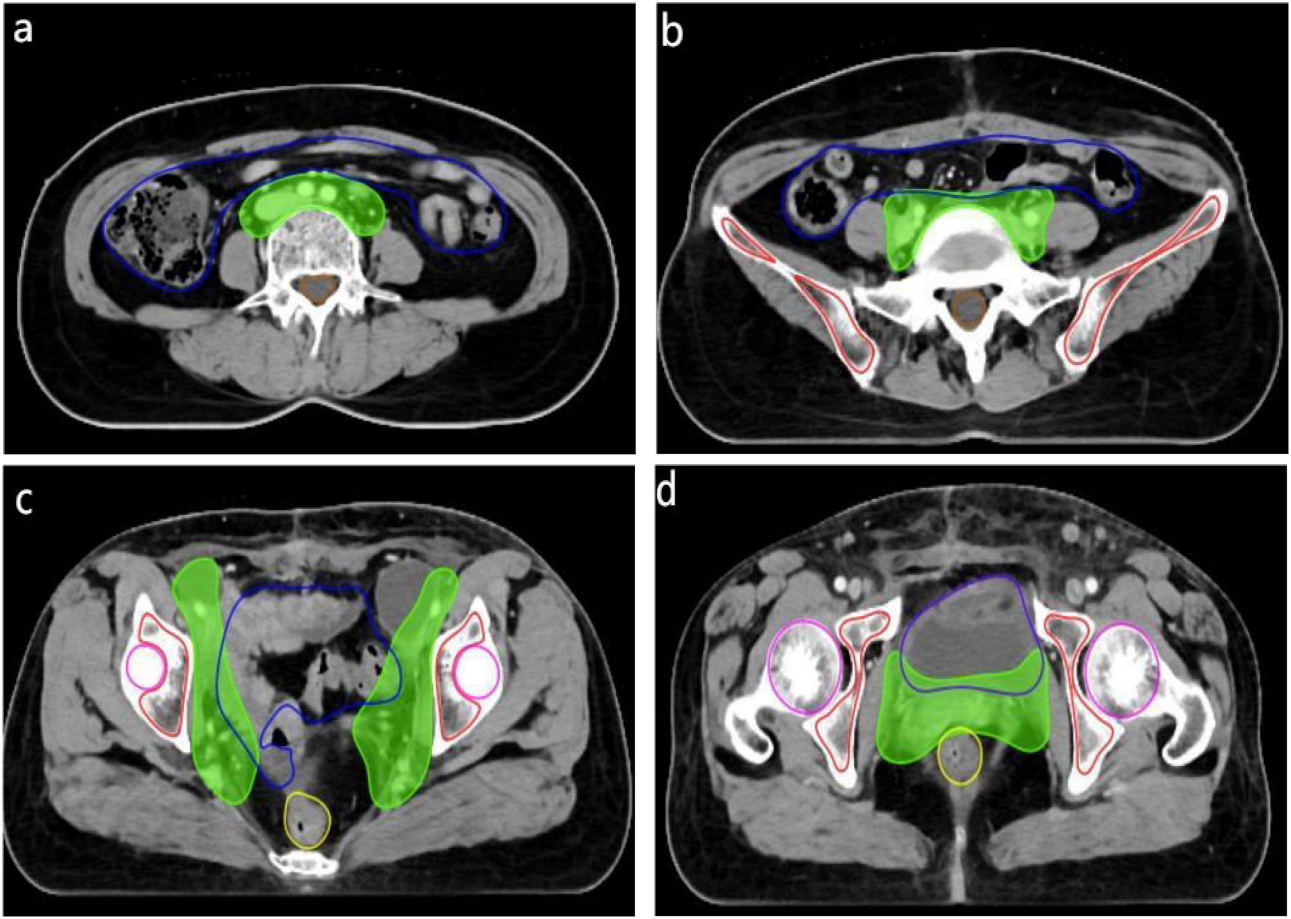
Representative axial CT images of the abdomen and pelvis at different anatomical levels: (a) axial CT image at the level immediately inferior to the kidneys (infrarenal level); (b) axial CT image at the pelvic inlet (iliac level); (c) axial CT image at the level of the true pelvis (acetabular level); (d) axial CT image at the lower pelvis, at the level of the urinary bladder and femoral heads.
